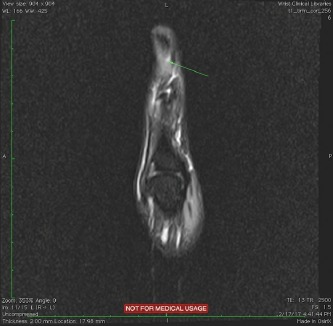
MRI appearance of the tumor – radial side view of the thumb
